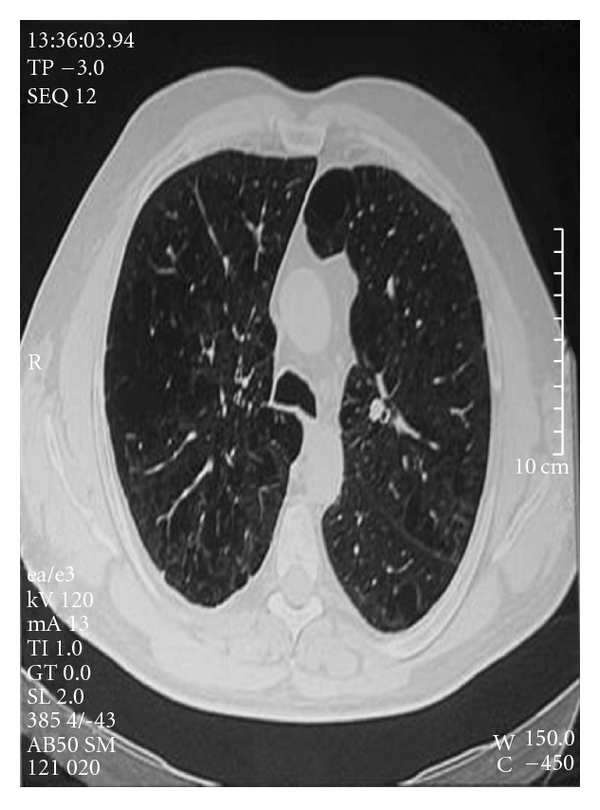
Two years before his admission he was presented with dyspnea on exception, and on CT imaging emphysema was the main evidence.
